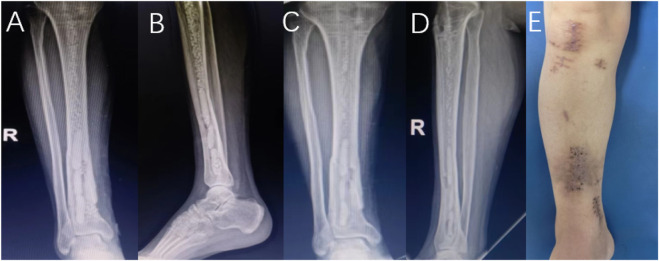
Male patient, 36 years old, no obvious abnormal exudation occurred within 4 weeks after the operation. X-ray (A,B) 1 day after antibiotic-loaded calcium sulfate implantation, X-ray (C,D) 1 month after the operation, and limb appearance (E) 4 weeks after the operation.
